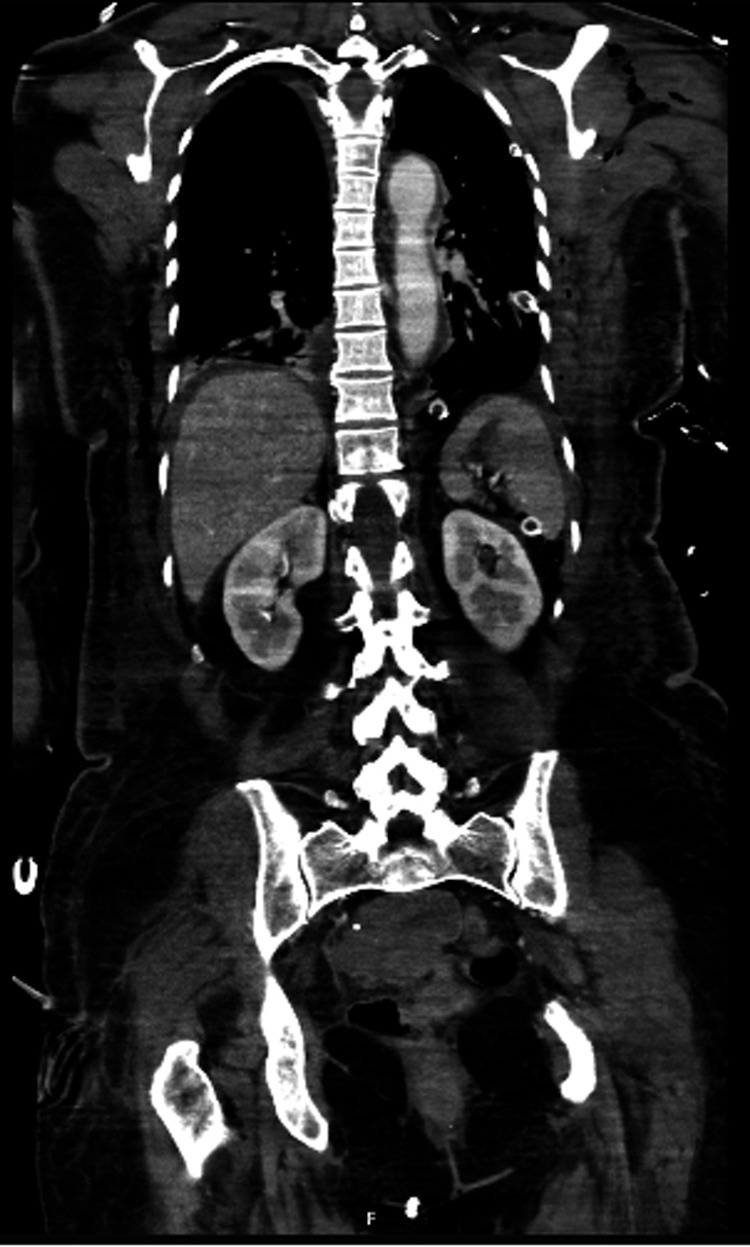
Post-operative non-contrast thoraco-abdomino-pelvic computed tomography
